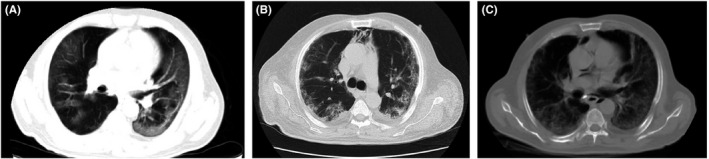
Chest CT scans of case 1
